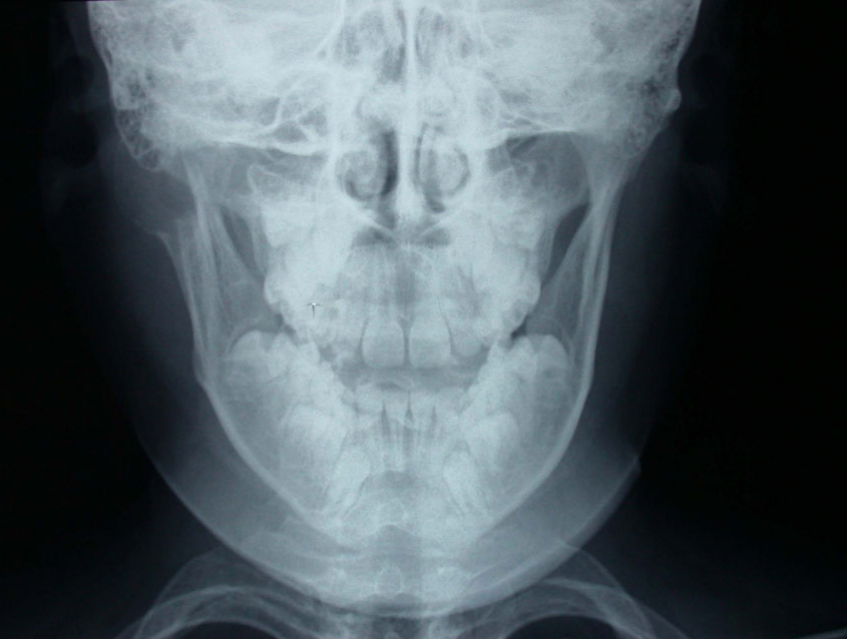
Posterior-anterior radiography of the skull. Radiolucent lesion of the right condyle.
